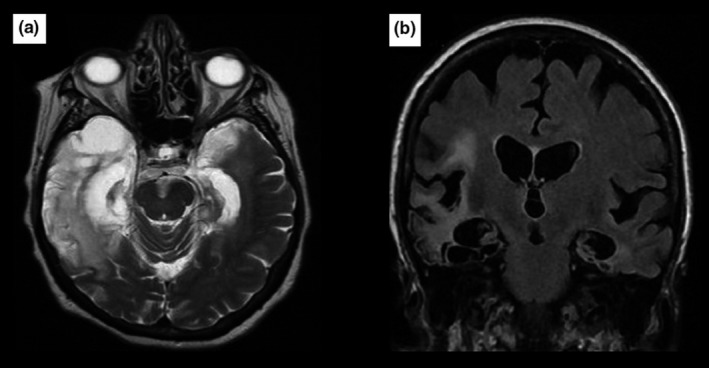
It is a 65‐year‐old man without no past medical history, admitted for the febrile confusional syndrome. *Brain MRI* shows on T2‐weighted images (a) and FLAIR sequences (b) bilateral hyperintensity in temporo‐insular regions predominant on the right. Diagnosis of herpetic LE was made after the biological investigations
